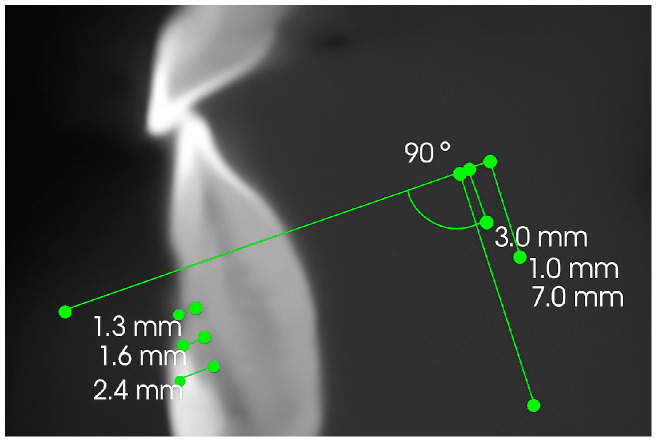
Measurements of marginal bone stability on CBCT.
